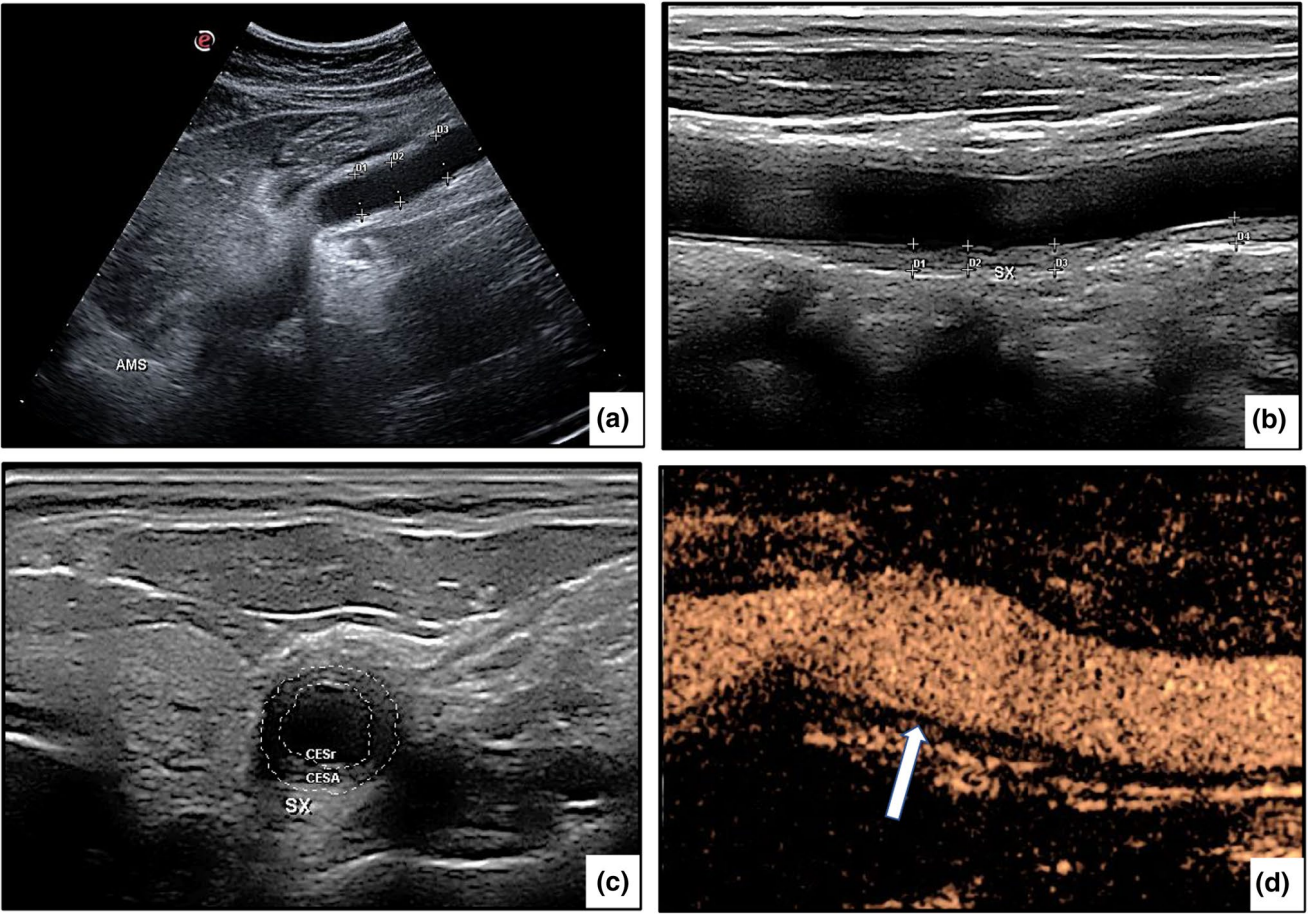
a A 33-year-old woman with type III Takayasu arteritis complained of abdominal pain, nausea, vomiting, and diarrhea. A B-mode ultrasound showed the rare finding of superior mesenteric artery aneurysm. The diagnosis of “superior mesenteric artery syndrome” was considered. b B-mode ultrasound image of the right common carotid artery in a 27-year-old man diagnosed with type IIa Takayasu arteritis. Longitudinal B-mode ultrasound shows mid-echoic, homogeneous circumferential wall thickening (“macaroni sign”). The thickness of the intima–media is between 1.3 and 2.0 mm (normal value ≤ 0.9 mm). c A transverse scan of the same vessel shows marked, concentric, hypoechoic wall thickening that reduces the lumen by > 50%. d Contrast-enhanced ultrasound of the carotid artery allows to detect a high uptake of microbubbles, indicating active disease
